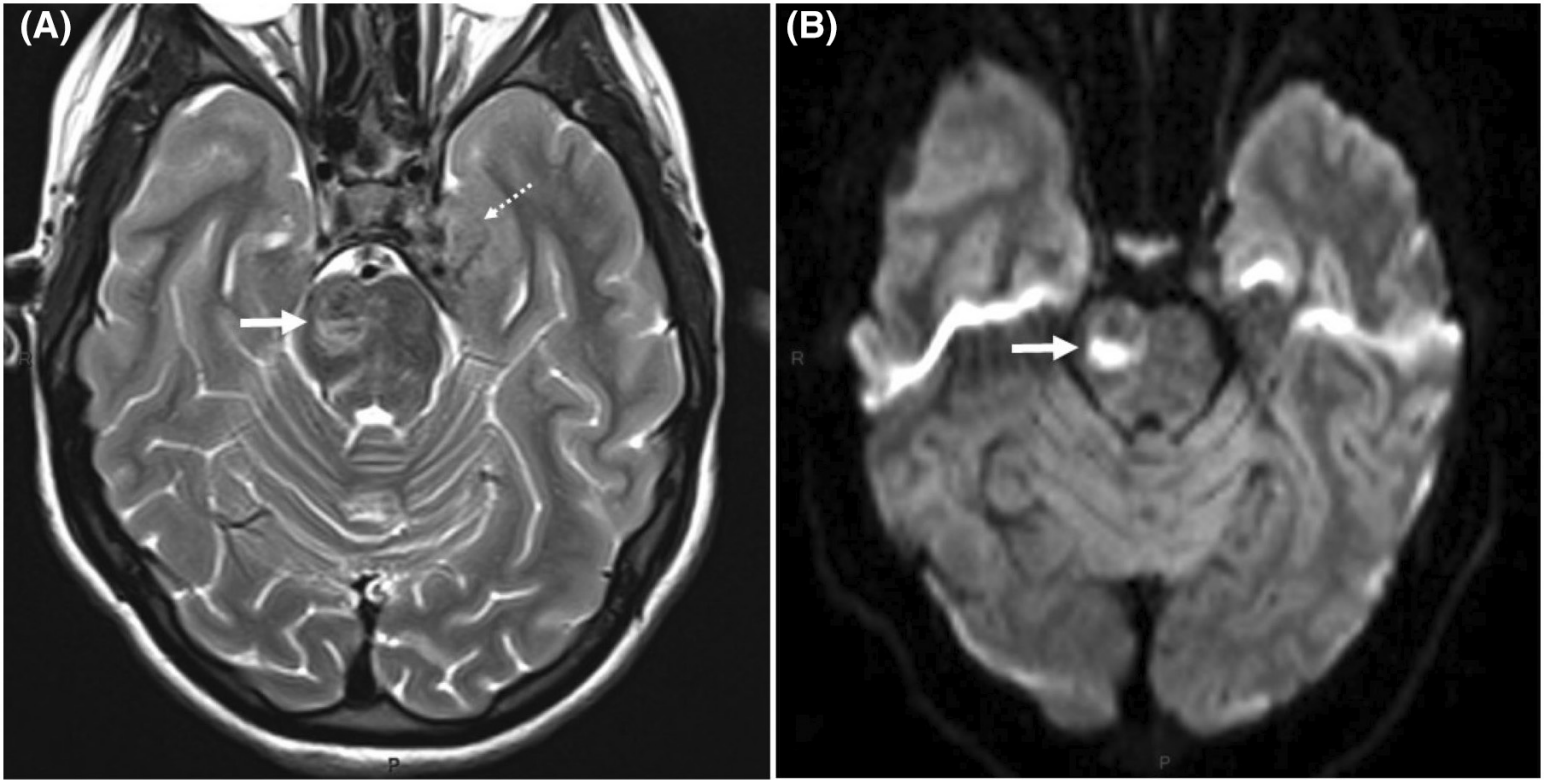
MRI work‐up at admission. Two axial transverse slices in similar slice location. (A) T2‐weighted (T2‐W) view shows heterogeneous parenchymal damage (arrow) of the right side of the ponto‐mesencephalic junction displaying with high signal intensity of its lower half which contrasts with low signal of its upper half. Partial volume on a schwannoma of the left Vth cranial nerve (dotted arrow). (B) DW view confirms the duality of the low/high signal intensities within the lesion (arrow) with high signal intensity of the lower half featuring cytotoxic edema.
